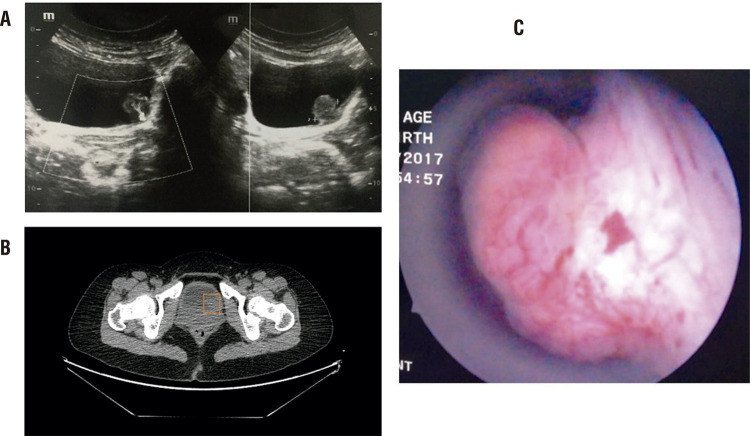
Imaging and cystoscopy. A) Ultrasound examination showing a hypoechoic mass with abundant blood flow inside in the urinary bladder. B) CT scan showing a polypoid mass (box) in the left wall of the urinary bladder. C) Cystoscopy showing solitary and broad basal neoplasms covered by smooth vesical mucosa in the left anterior wall of the urinary bladder.
